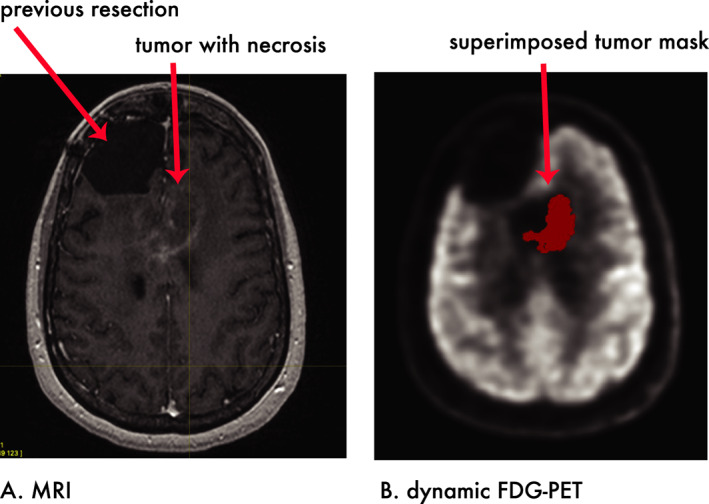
Dynamic FDG‐PET in brain tumor treatment. (A) MRI provides anatomic structure of the tumor which, (B) when co‐registered upon dynamic FDG‐PET with the locations and shapes of tumors, provides local measures of glucose kinetics within each tumor. Preliminary work established that tumor‐site‐associated glucose kinetics can help create statistical models that better differentiate tumor progression from radiation‐induced inflammation.
